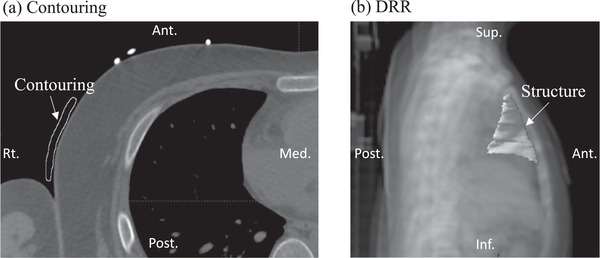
Contouring of compensating material in a treatment planning system (TPS) and digitally reconstructed radiograph (DRR) with soft variable shape tungsten rubber (STR). (a) Contouring of compensating material in TPS and (b) DRR with STR
